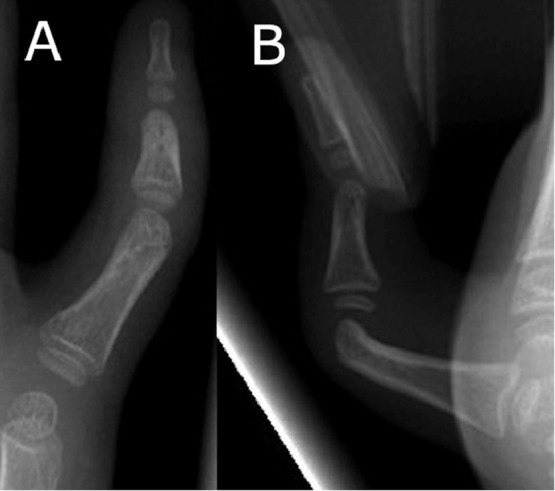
A plain x-ray identified no bony abnormality. The involved PIP joint is held in flexion (A) = Anterior posterior (B) = Lateral.
